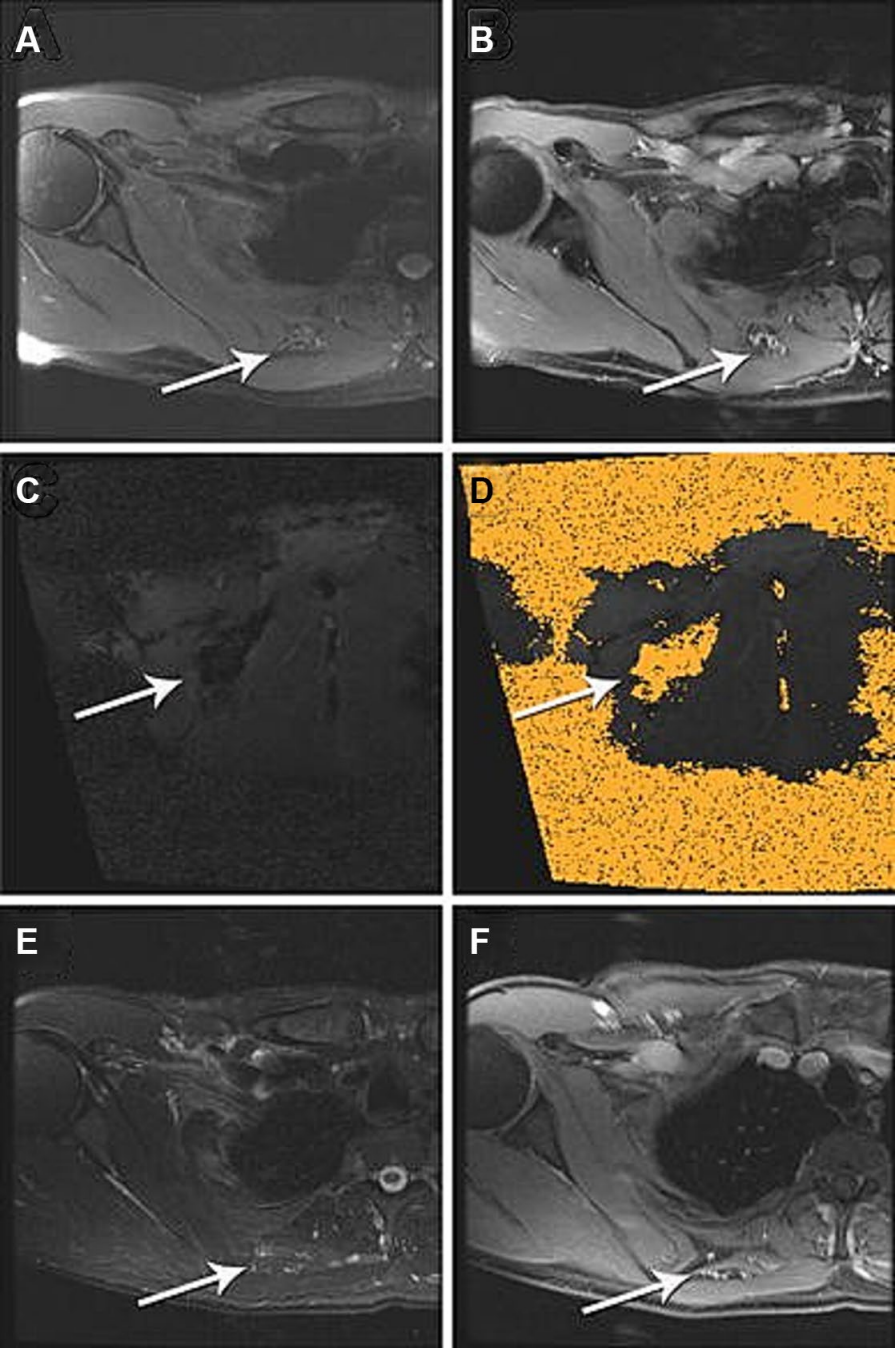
IMRI laser ablation of a LFVM in the soft tissue between the right rhomboid and subscapularis muscles. Pre-ablation (A) axial T2-weighted and (B) gadolinium-enhanced axial T1-weighted spoiled gradient echo (SPGR) MRI demonstrate heterogeneous abnormal T2 signal [(A) white arrow] and contrast enhancement [(B) white arrow] within the small vascular anomaly. (C) Intra-procedural coronal oblique phase imaging demonstrates real-time tissue heating using proton resonance frequency MR thermometry and (D) thermal damage map calculated from this using the Arrhenius equation to estimate the ablation zone. (E) One-year post-ablation axial T2-weighted MRI demonstrate no significant T2 signal (white arrow). (F) Gadolinium-enhanced axial T1-weight spoiled gradient echo (SPGR) MRI and no enhancement of the vascular anomaly (white arrow). Reproduced with permission from Thompson et al. (23).
